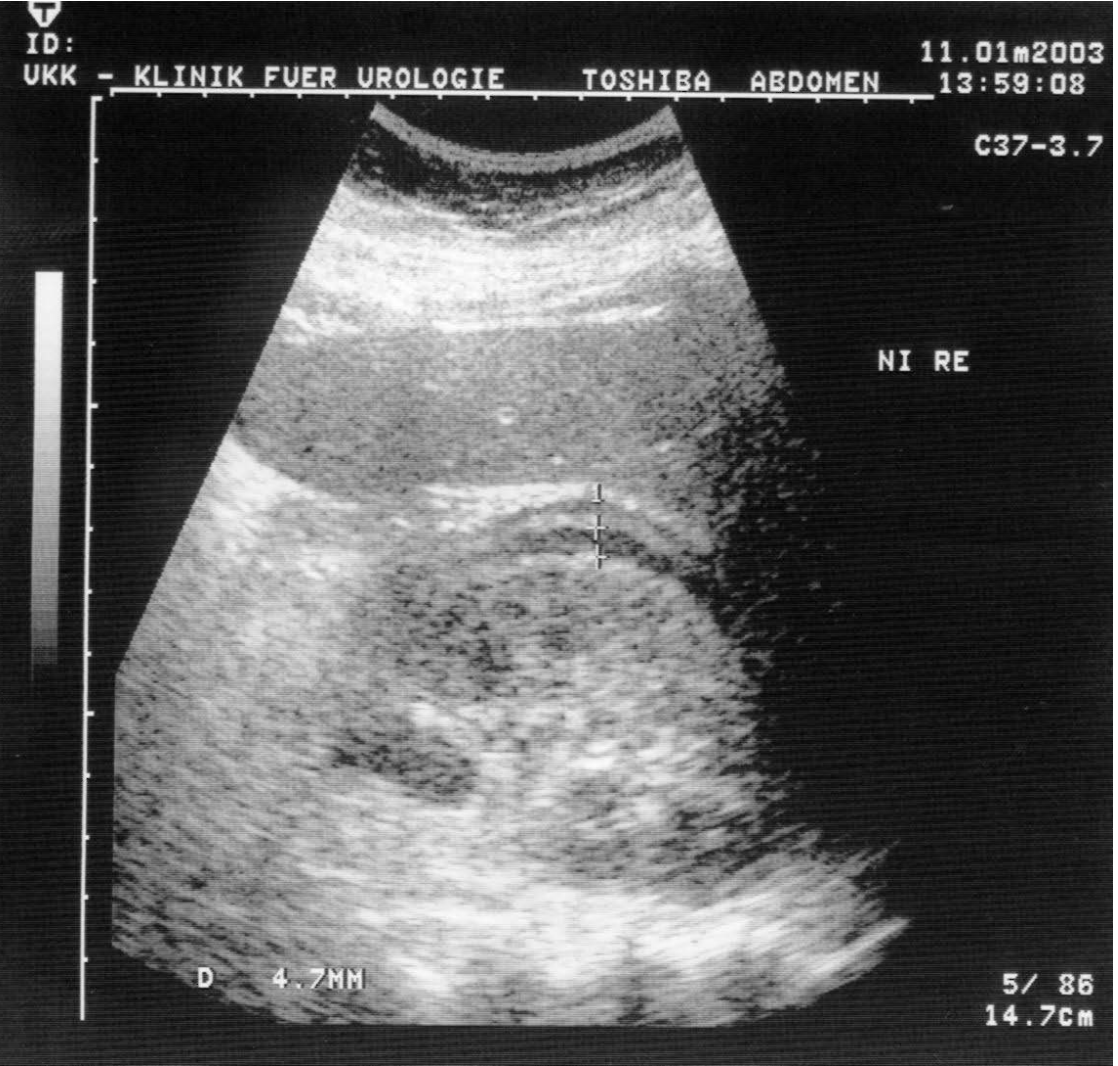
Ultrasound image of the right kidney with perirenal fluid extravasation within the Gerota's fascia.
